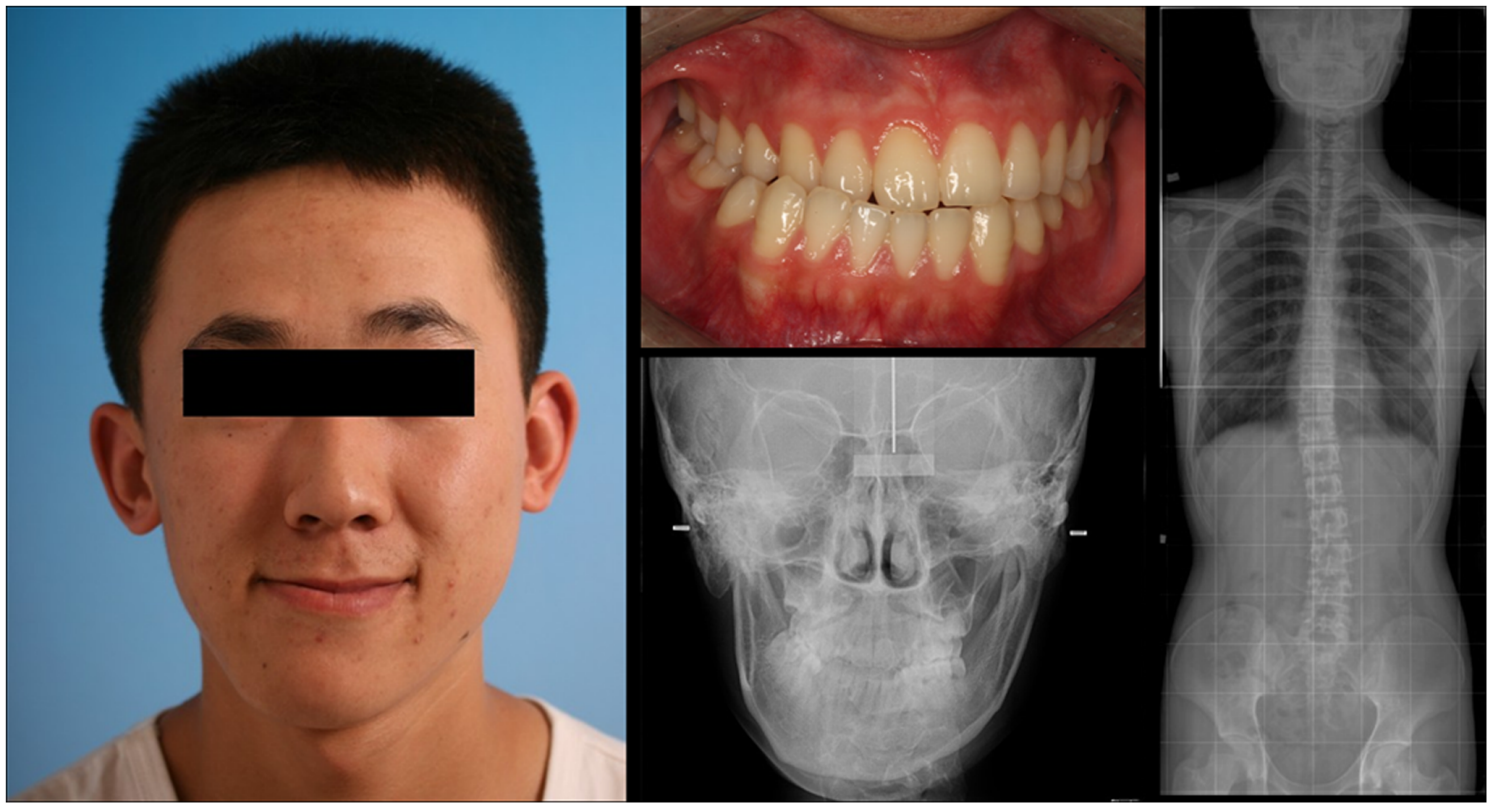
Image from a typical patient with mandibular deviation. Anterior X-ray film. The lengths of the mandibular rami have an obvious difference. The vertical distance from the chin ridge to the inferior orbital fissure line is greater than 2 mm.
